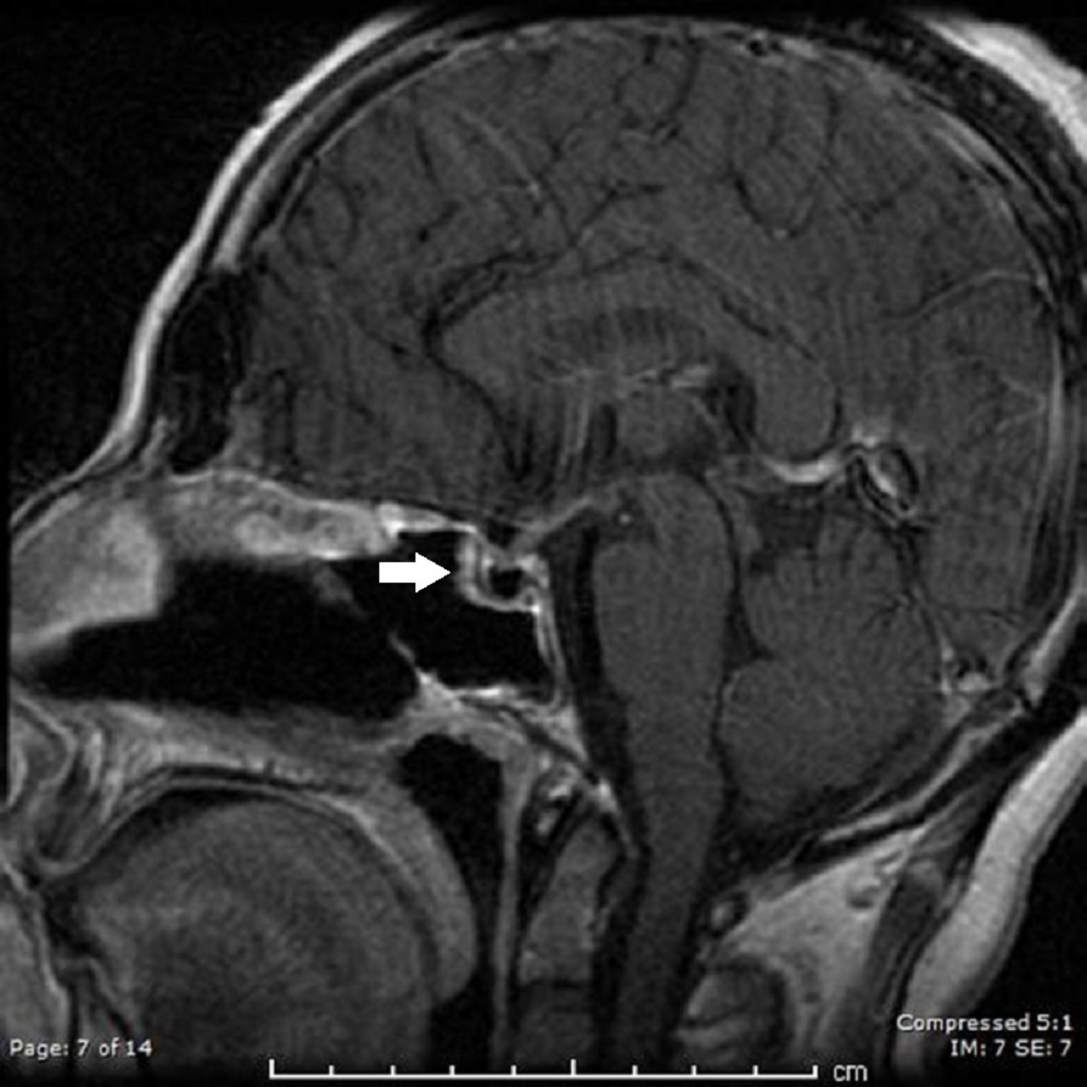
Post-contrast sagittal T1 magnetic resonance imaging after surgical extirpation of cyst
